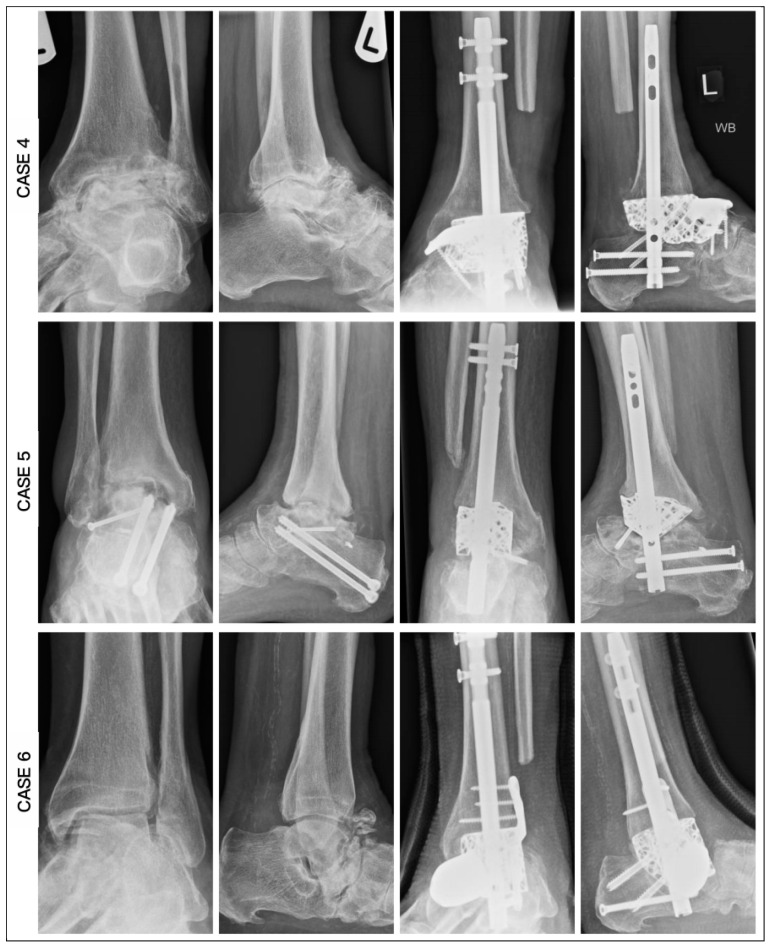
Plain radiographs of AP and lateral pre- and post-fixation for cases 4 to 6.
